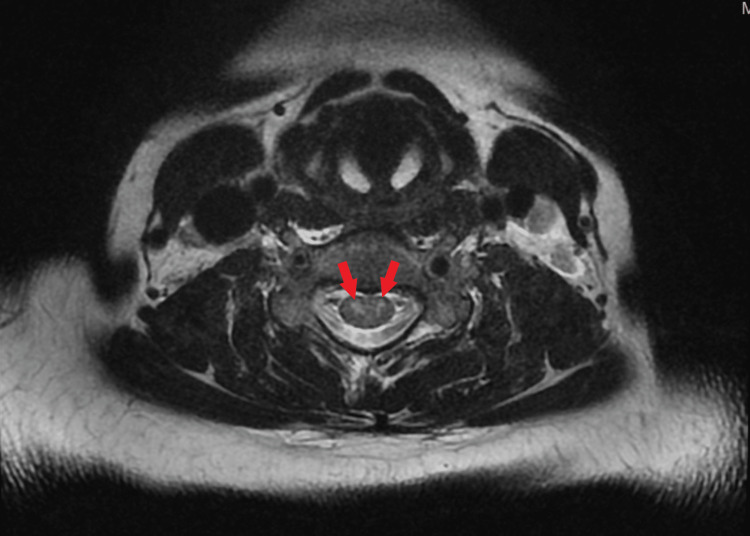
MRI of the cervical spine (axial T2 view) showing bilateral high T2 signal intensity known as 'Owl's eyes'
